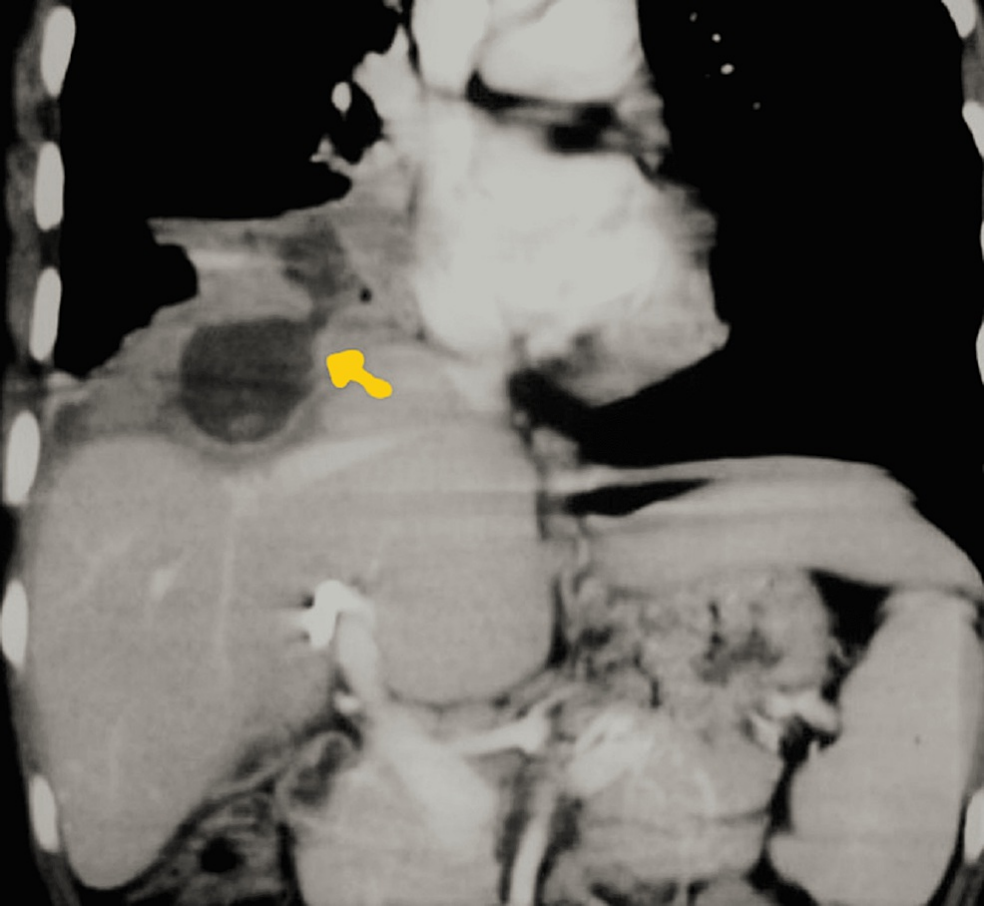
CT abdomen showing bronchobilliary fistula.
